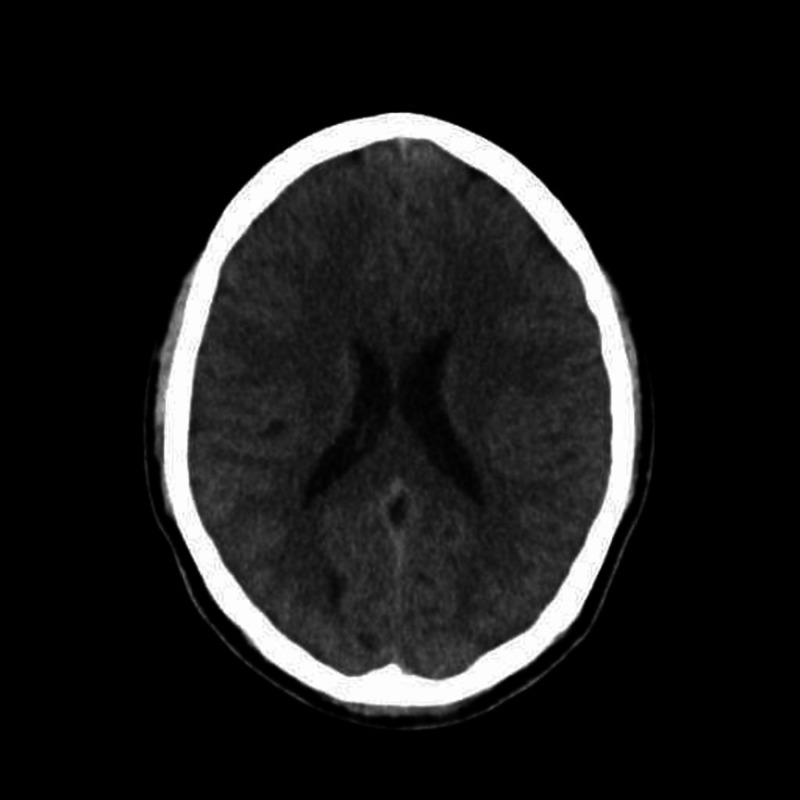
CT scan of head in axial view This is a normal CT scan of the head.
